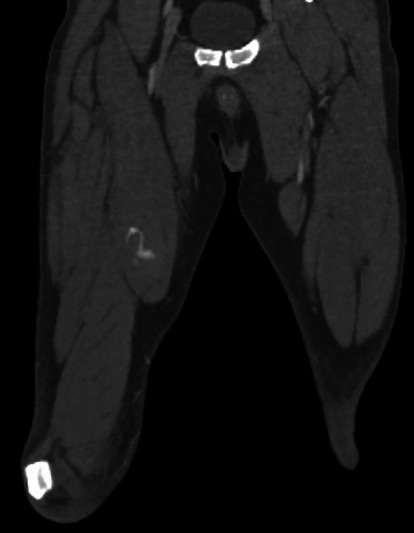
A coronal CTA image of bilateral thighs demonstrating a hematoma in the sartorius of the right thigh.
